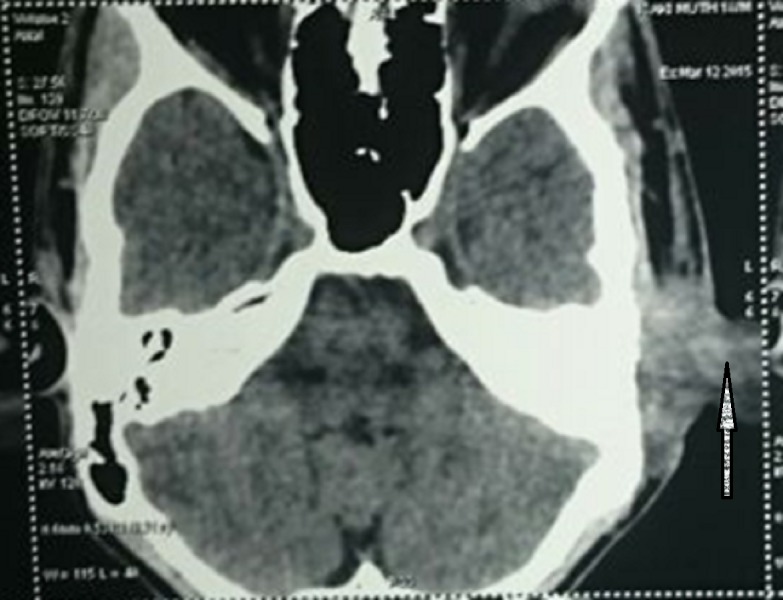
CT of the temporal bone showing a space-occupying lesion at the left post-auricular region CT - Computed tomography.
